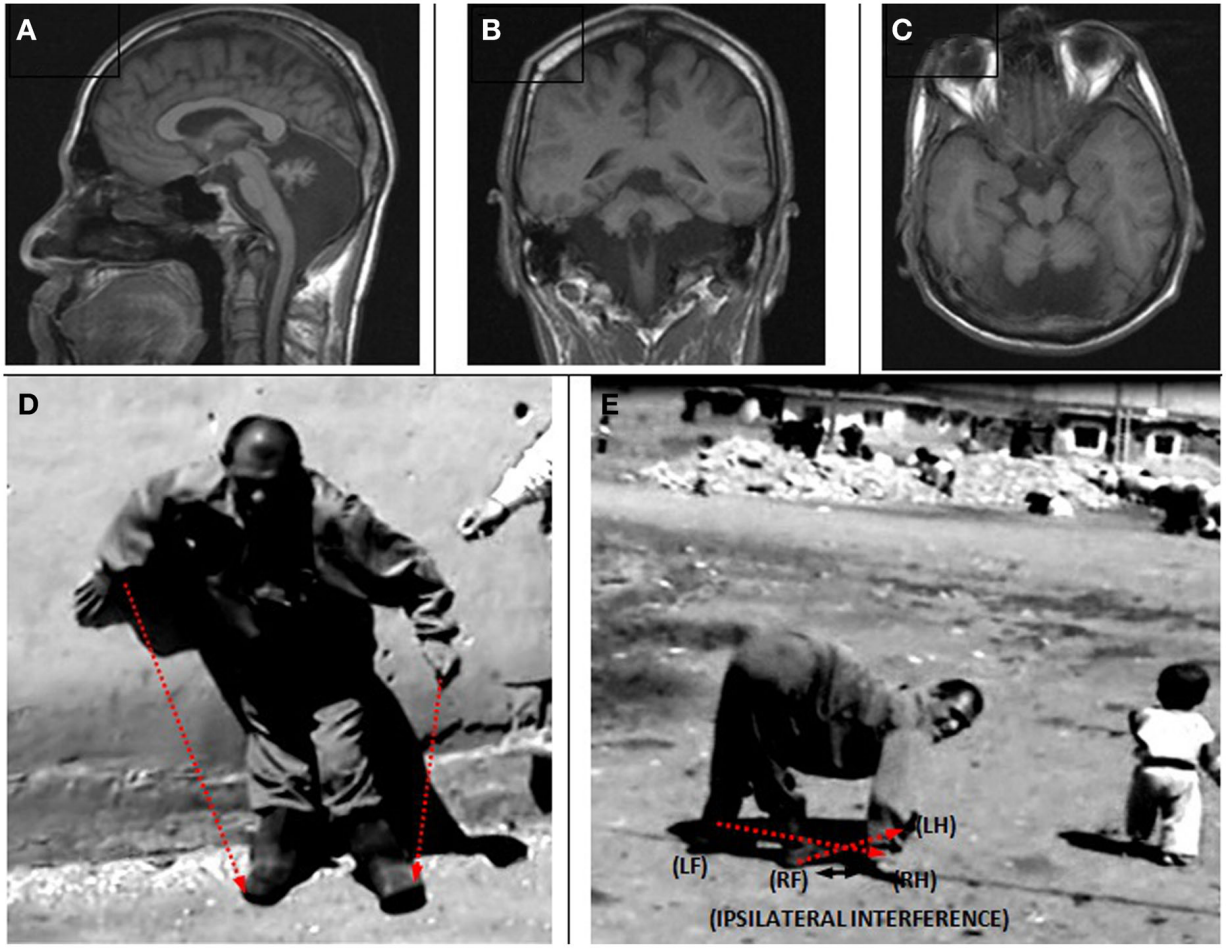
Case IV-22. Sagittal (A), coronal (B) and axial (C) MRI scans. (D) Releasing from the wall to make a step: notice the parallel action of the left arm and left leg; (E) diagonal-sequence QL soon after releasing from the wall: notice the interference between the right arm and right leg.
